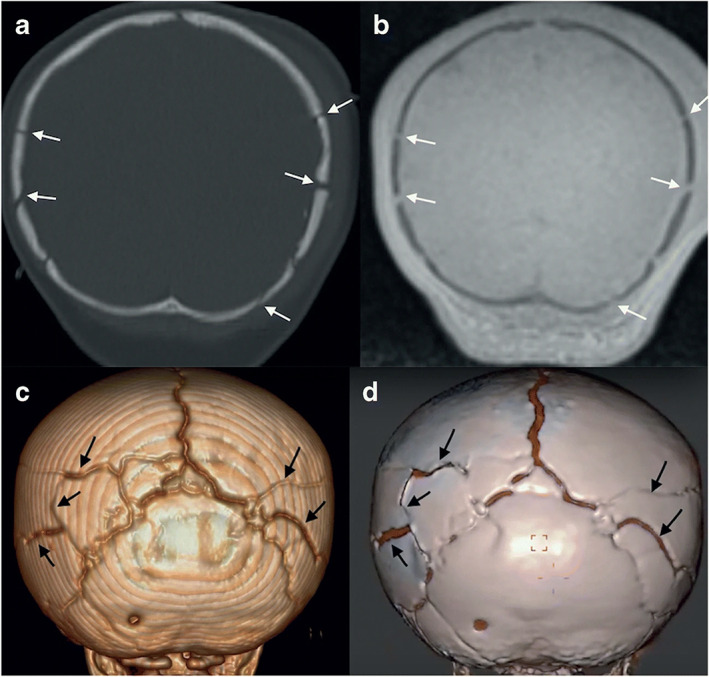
A 9‐month‐old with multiple skull fractures (arrows) demonstrated on coronal head computed tomography (CT) (a), and coronal black bone magnetic resonance imaging (MRI) (b) and the corresponding 3D rendering (c, d). Reprinted by permission from Springer Nature from reference 70.
